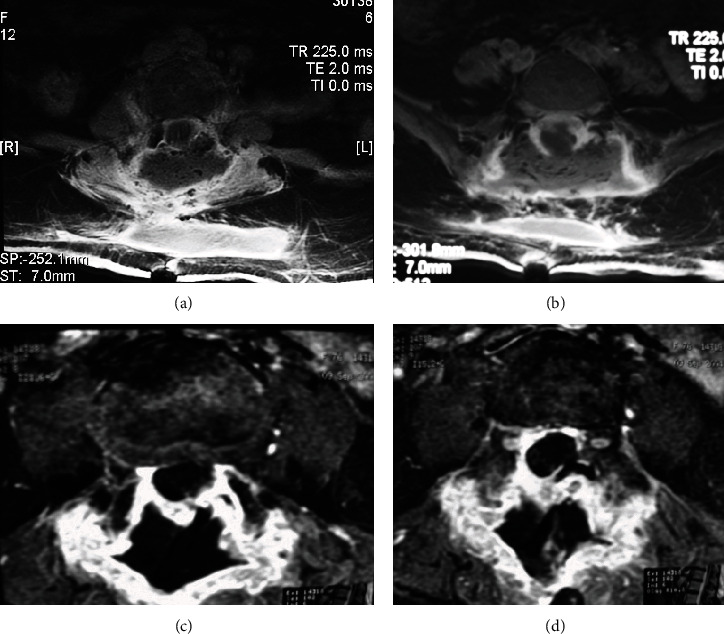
(a–d) Postgadolinium MRI is showing abscesses at the lumbar spine. (a, b) Serosanguineous fluid collections extend to the subcutaneous tissue. (c, d) Intense enhancement of the dural sac.
